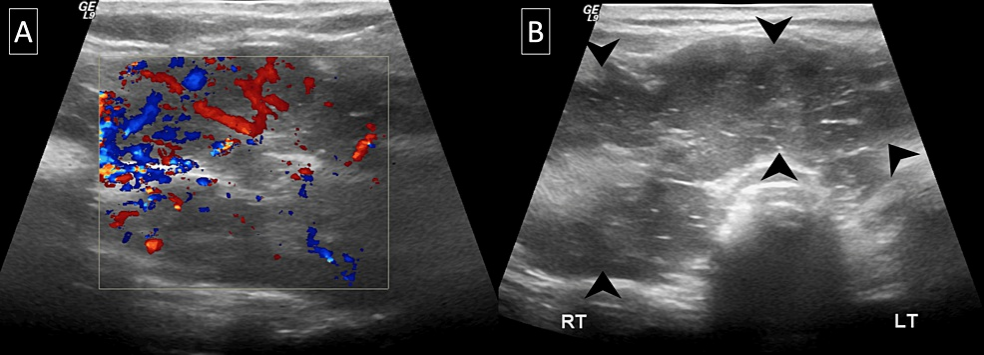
Thyroid ultrasound. Initial thyroid color Doppler (A) and grayscale (B) ultrasound images demonstrate a heterogeneous thyroid gland with an enlarged and hypervascular right thyroid lobe/isthmus (arrowheads, B).
